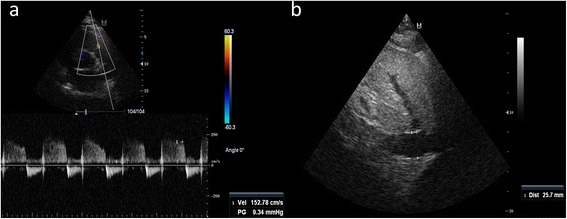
Example for estimating the pulmonary artery diastolic pressure (PAdP). a. The end-diastolic velocity of the pulmonary regurgitation jet was measured (parasternal short-axis view) and pressure gradient was automatically calculated (9.34 mmHg). b. Right atrial pressure was estimated in 15 mmHg through the analysis of size (dilated, 25.7 mm) and collapsibility (diminished) of the inferior vena cava. PAdP is obtained as the sum of 9.34 and 15 mmHg, resulting in a highly elevated PAdP (equivalent to an elevated left atrial pressure), of 24.3 mmHg
